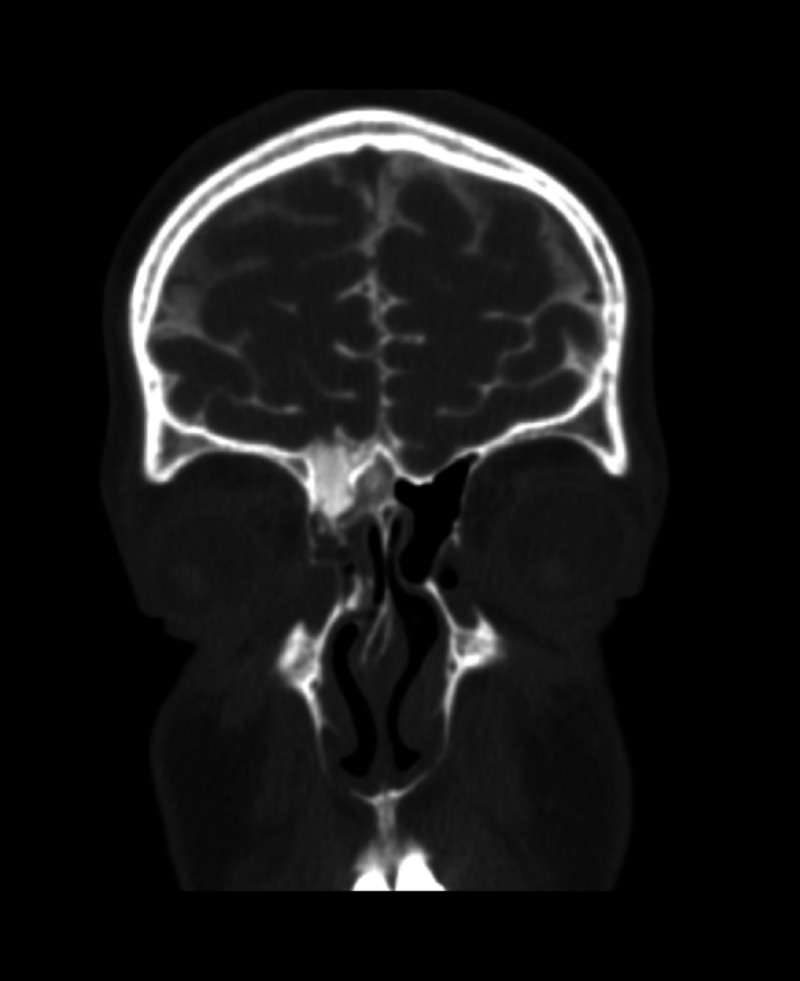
CT cisternography showing a frontal cerebrospinal fluid leak. CT: computed tomography.
